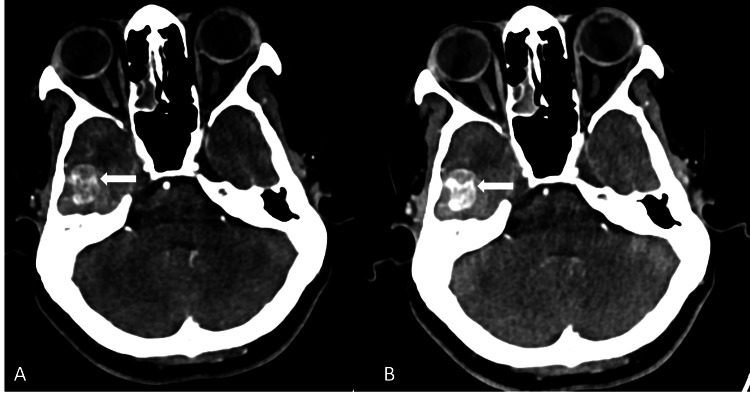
(A, B) Axial contrast-enhanced CT images in arterial and venous phases demonstrate intense heterogeneous enhancement of the right temporal extra-axial lesion with progressive increase in enhancement in the venous phase (arrows).
